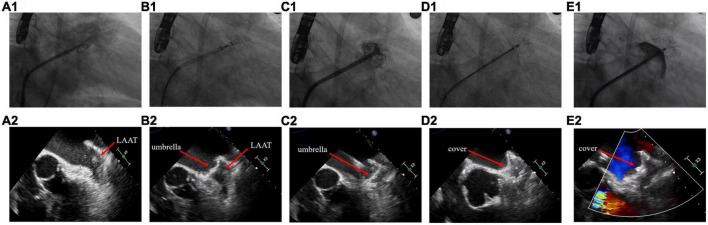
Left atrial appendage closure strategy in patients with left atrial appendage thrombus. (A) Fluoroscopy and transesophageal echocardiography images before the procedure. (B) The umbrella of the LAmbre device was partially deployed at the ostium of left atrial appendage (LAA). (C) The umbrella was propelled distally to the LAA and expanded. (D) The cover was deployed to seal the LAA ostium. (E) Detection of peridevice leak.
